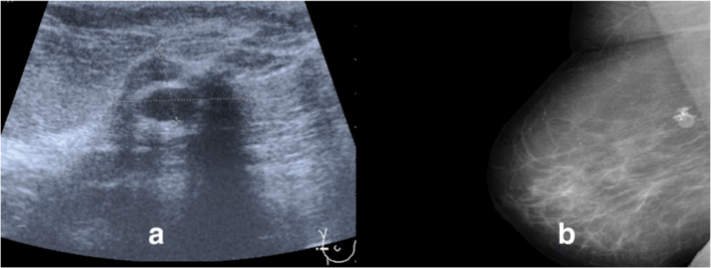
a Sonographic picture of the breast showing an irregular lesion, 11.1 × 20.1 mm. b Mammography showed a calcified prepectoral fine delimited mass
